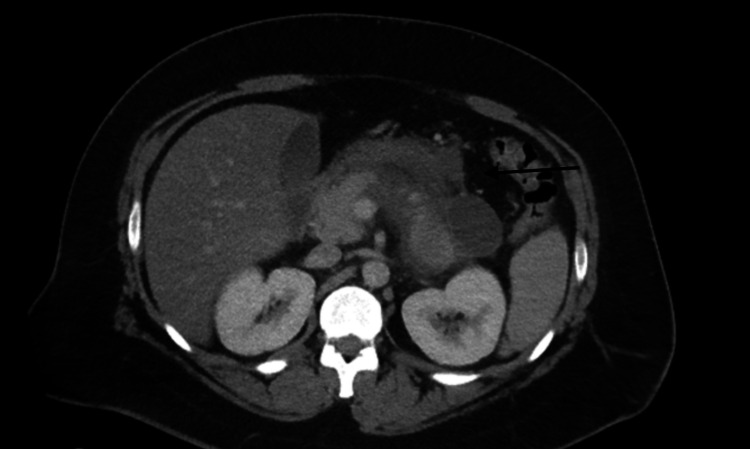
CT scan of the abdomen without contrast revealed acute interstitial edematous pancreatitis primarily involving the pancreas body and tail.
